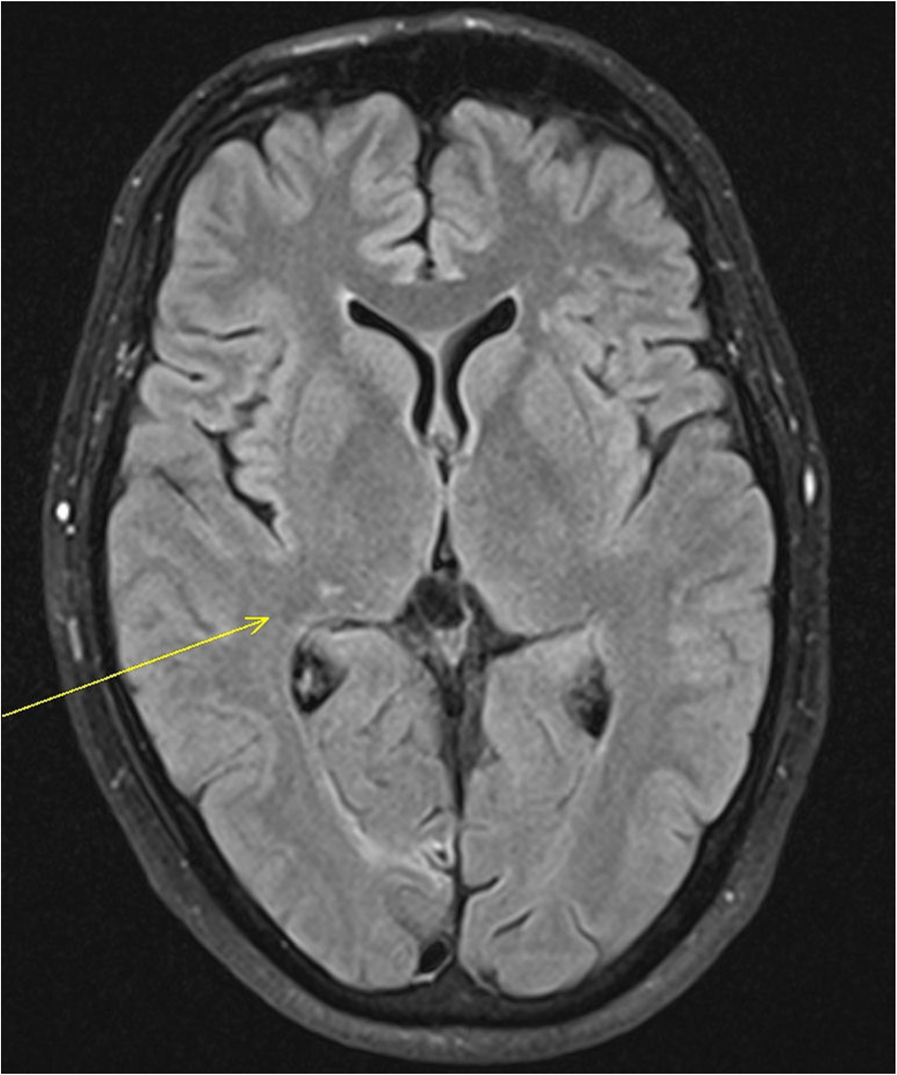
Axial Magnetic resonance Imaging (MRI) of the Brain with Fluid Attenuated Inversion Recovery (FLAIR sequence), three months after completion of chemotherapy: Hyperintense lesion in the right thalamic region (arrow) indicating thalamic infarction.
